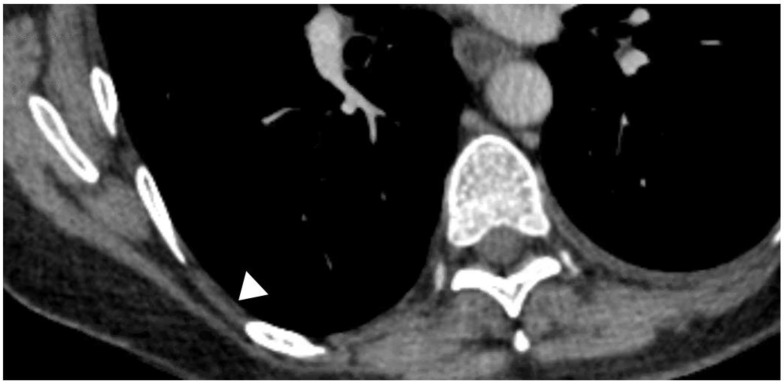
CT appearances of the “pleural stripe”; (white arrow head)
